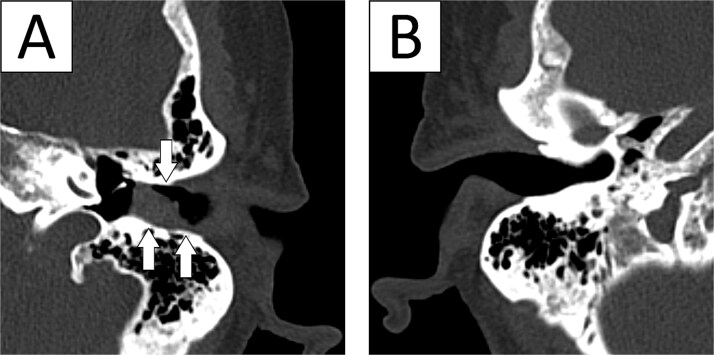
Non-contrast head CT in case 1. (A) Affected left ear demonstrating hyperdense blood within the external auditory canal at the level of the tympanic membrane, with adjacent soft-tissue blood products near the auricular/mastoid region (arrows). (B) Contralateral non-affected ear without comparable abnormality.
